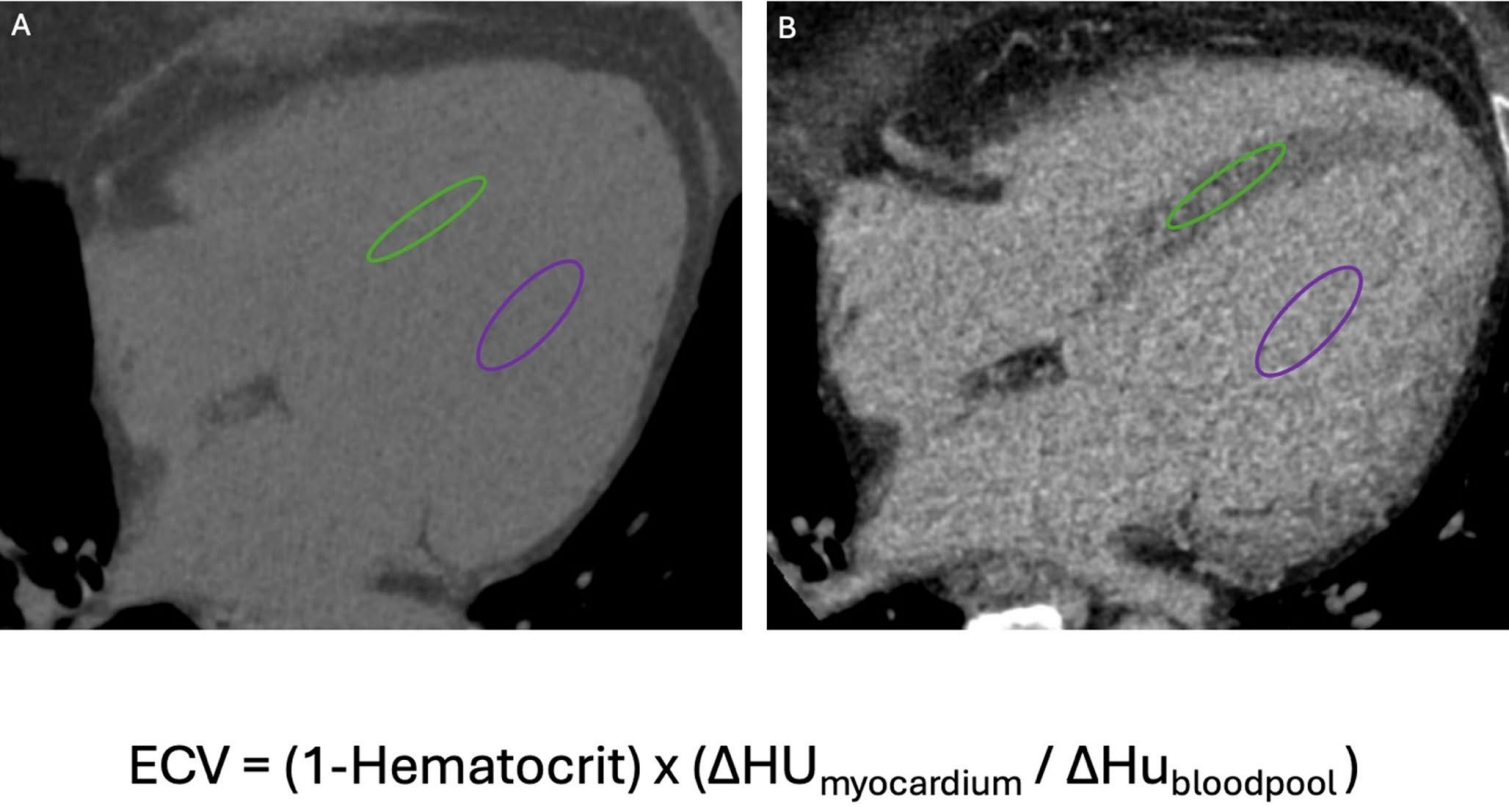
Extracellular volume (ECV) quantification using computed tomography. The calculation of ECV is based on the ratio between the concentration of contrast agent in the myocardium and in the plasma component of the blood pool. Therefore, measuring the patient’s hematocrit is essential to convert whole blood concentrations into plasma concentrations. Hounsfield unit (HU) measurements are obtained by placing regions of interest (ROIs) in the left ventricular cavity (for blood pool values, purple ellipse in Panels A and B) and in the myocardium (green ellipse in Panels A and B), carefully avoiding papillary muscles and trabeculae. This allows for the assessment of extracellular contrast agent uptake in both pre-contrast (Panel A) and post-contrast (Panel B) scans. The differences in HU (∆HU) between pre- and post-contrast images are then used to evaluate the distribution of contrast agent, according to the formula shown in the lower panel
